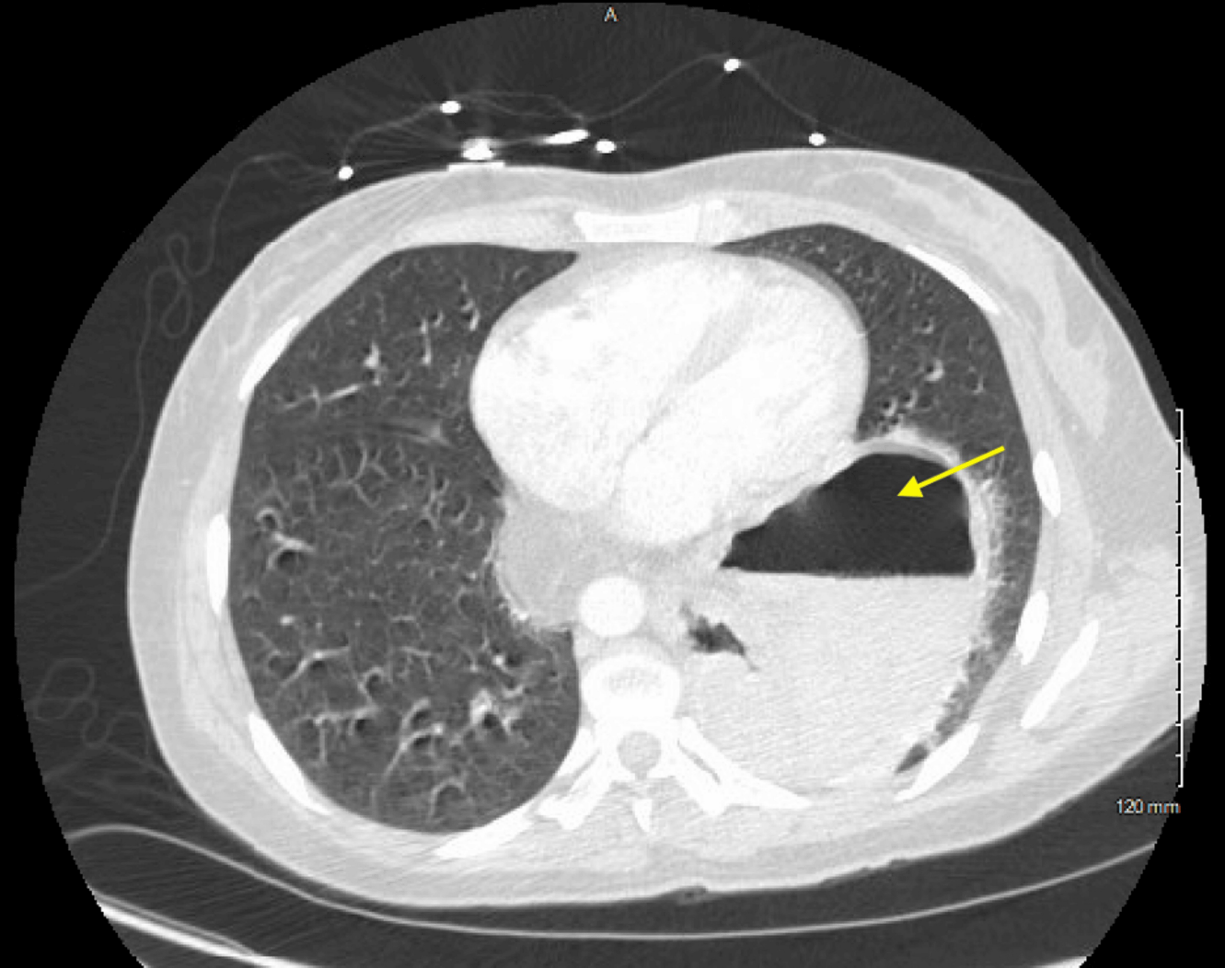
Axial cross-sectional CT scan of the abdomen and pelvis reveals a large diaphragmatic hernia at the hiatus. The stomach is quite dilated, with a prominent air-fluid level. Pylorus and the distal antrum are attenuated as they extend through the defect into the thorax (yellow arrow)
